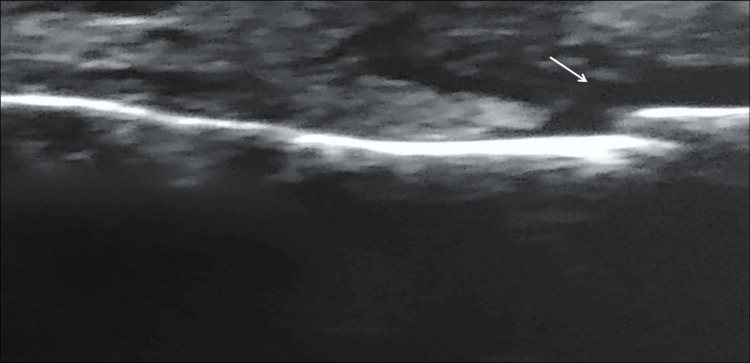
High-resolution ultrasonography of the displaced nasal bone fracture The ultrasound image corresponds to the same injury shown in the CT scan (Figure 1), demonstrating the step-off deformity and cortical break (arrow). The acoustic pattern strongly correlates with the bony displacement visualized on CT.
